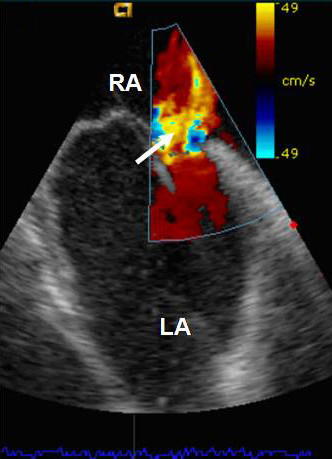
Image obtained using intracardiac echocardiography (ICE): With colour flow mapping (arrow) of the interatrial septum demonstrating a large ASD with shunting.
